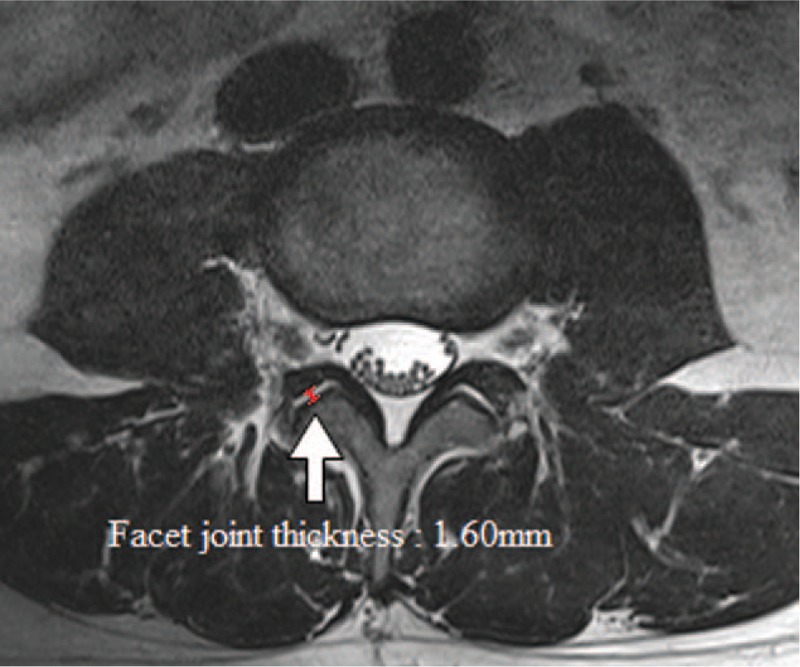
Measurement of the facet joint thickness on MRI at the L4-L5 level.
